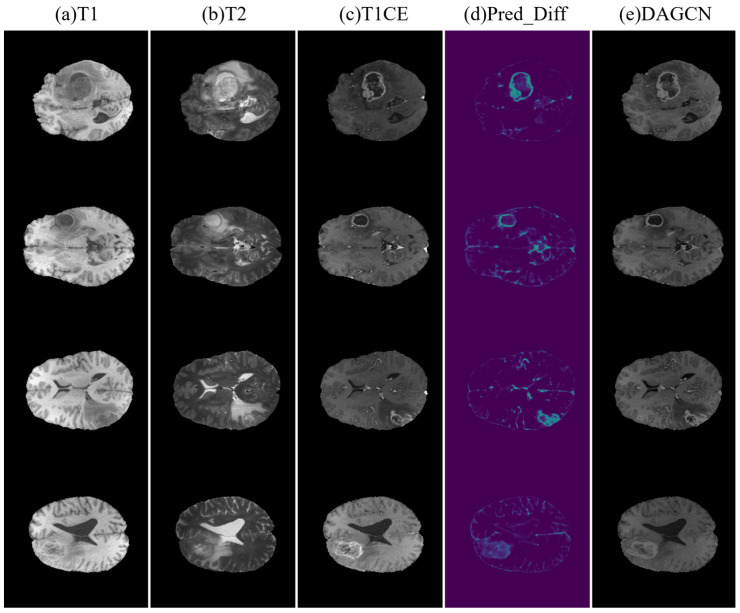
Representative generation process of DAGCN under T2-guided synthesis. For each case, the non-contrast T1-weighted images, auxiliary T2 image, predicted discrepancy map, synthesized T1-CE image, and ground-truth T1-CE image are shown for comparison. Although the predicted discrepancy maps generally localize the lesion region, residual interference from cerebrospinal fluid and nonspecific hyperintense areas may still be observed under T2 guidance. Predicted discrepancy maps are displayed using the perceptually uniform viridis sequential colormap with a consistent scale, following recommendations for scientific color use [42].
